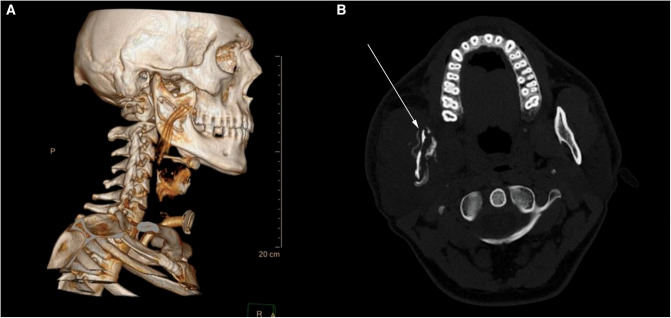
Axial CT scan of the neck at the C1 level after the relapse of the infection. (A) 3D reconstruction showing sequestrum of bone with a corrugated drain properly placed above the osteomyelitic site. (B) Cross section of the osteomyelitic mandibular ramus—bone is thin with loss of both medulla and cortex. A reconstructive plate was placed so the mandible could withstand masticatory forces.
